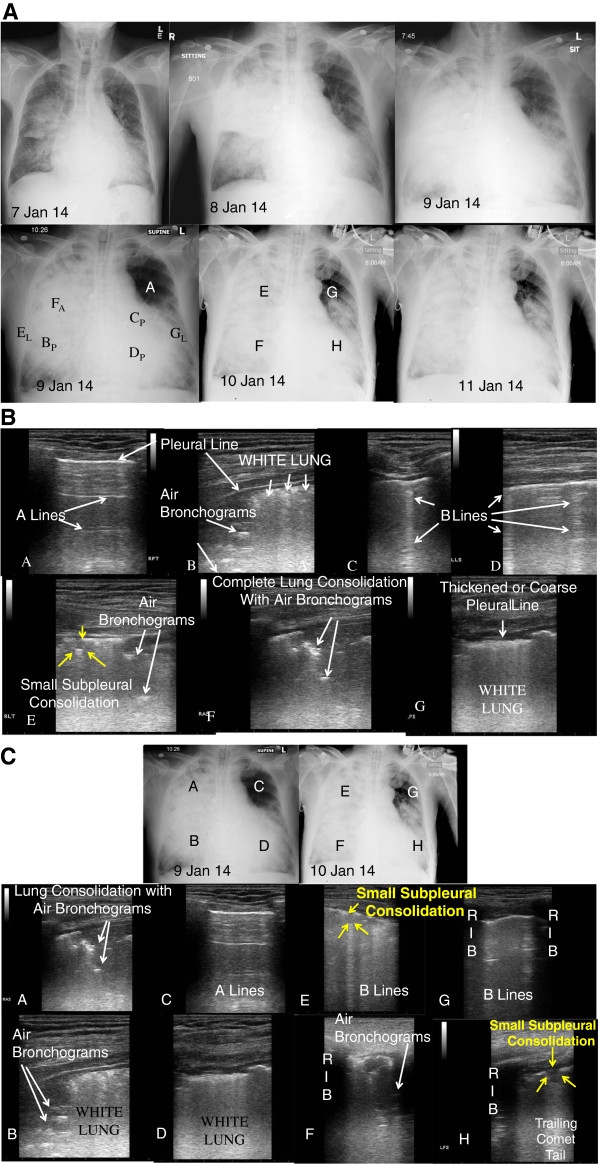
Radiography and ultrasonography for case 2. (A) Chest X-rays showing progression over course of illness. CXR Letters correspond to panel letters in Figure
